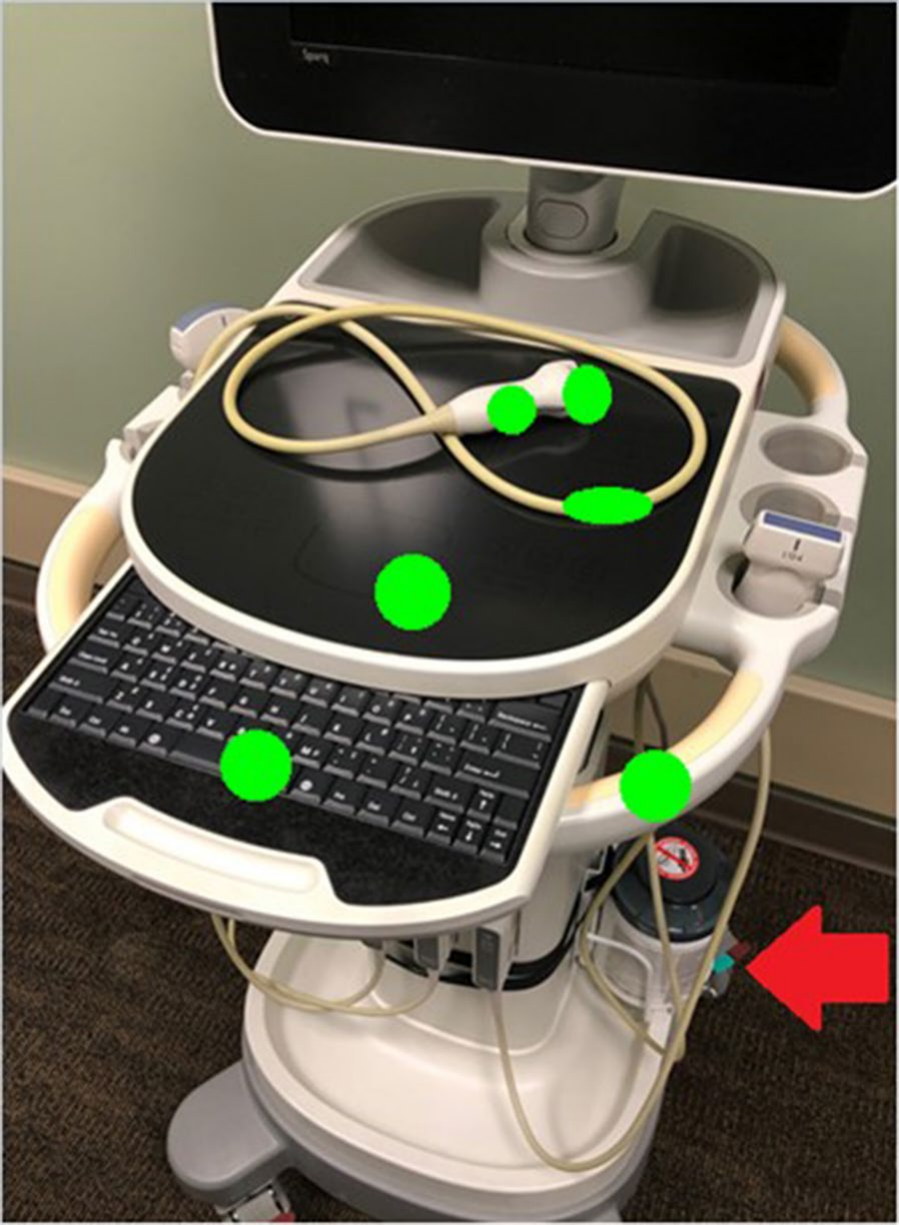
Locations of fluorescent marks. A Phillips SPARQ model ultrasound machine is pictured to demonstrate marking locations. Green circles represent the areas marked with fluorescent gel during the study (size exaggerated for clarity). For simplicity, only one probe and cord is displayed, but all probes and cords were marked in this fashion during the study. Also pictured is the disinfecting wipe caddie that was added to these models to improve access to cleaning supplies (red arrow)
